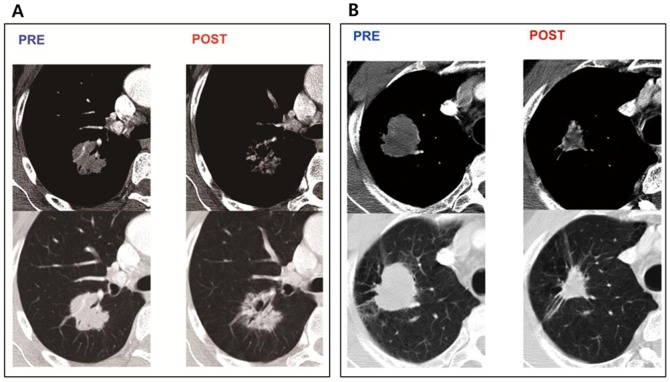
Quantitative CT parameters of pathologic responder. (a) Combined histogram distributions of the treatment before (blue) and after (red). As for histogram distribution, the vertical axis in each histogram shows the number of pixels in the segmented tumor. The horizontal axis shows the CT attenuation values. Attenuation value is shifted to the left from the blue to the red graph. (b) Intensity size-zone matrix of the treatment before (blue) and after (red). The horizontal axis shows size of homogenous area. Divergent distribution on the horizontal axis indicates increased size-zone variability. The vertical axis shows intensity. Divergent distribution on the vertical axis indicates increased intensity variability. Distributions of the points on the matrix become more convergent and clustered both in the horizontal axis and the vertical axis from the pre-treatment matrix to the post-treatment matrix.
